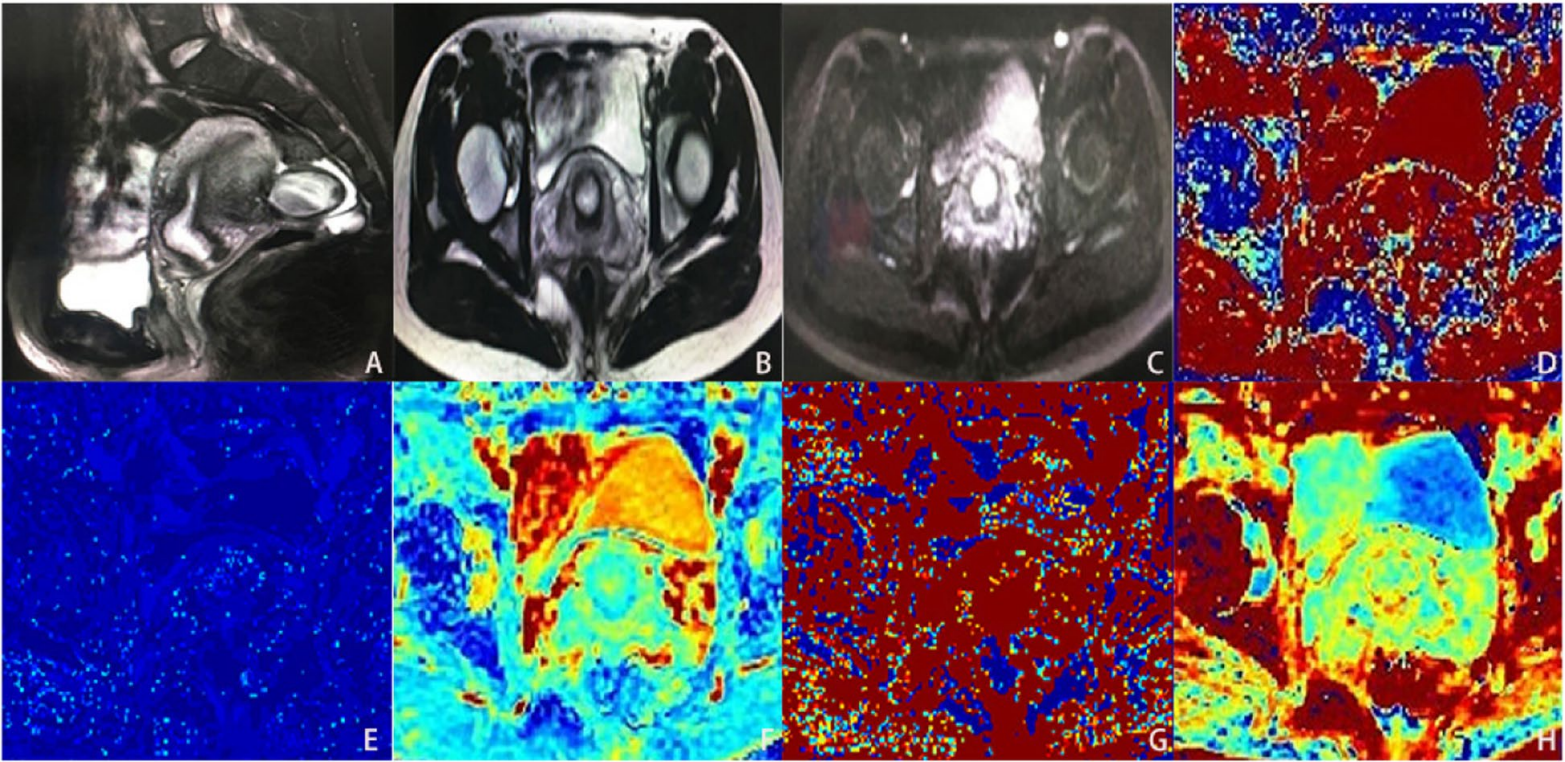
Normal cervical tissu. A is sagittal T2WI. B is axial T2WI. C is DWI (b = 800s / mm2). D is the pseudo color image of parameter D in IVIM. E is the pseudo color image of parameter D* in IVIM. F is the pseudo color image of parameter MD in DKI. G is the pseudo color image of parameter f in IVM. H is the pseudo color image of parameter MK in DKI
